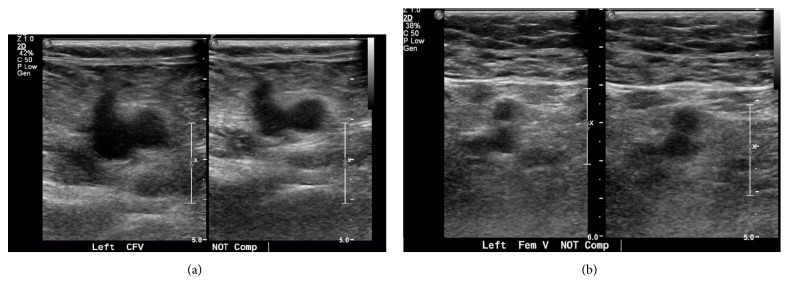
Doppler US of left lower limb showing DVT in the left common femoral (a) and femoral veins (b).
